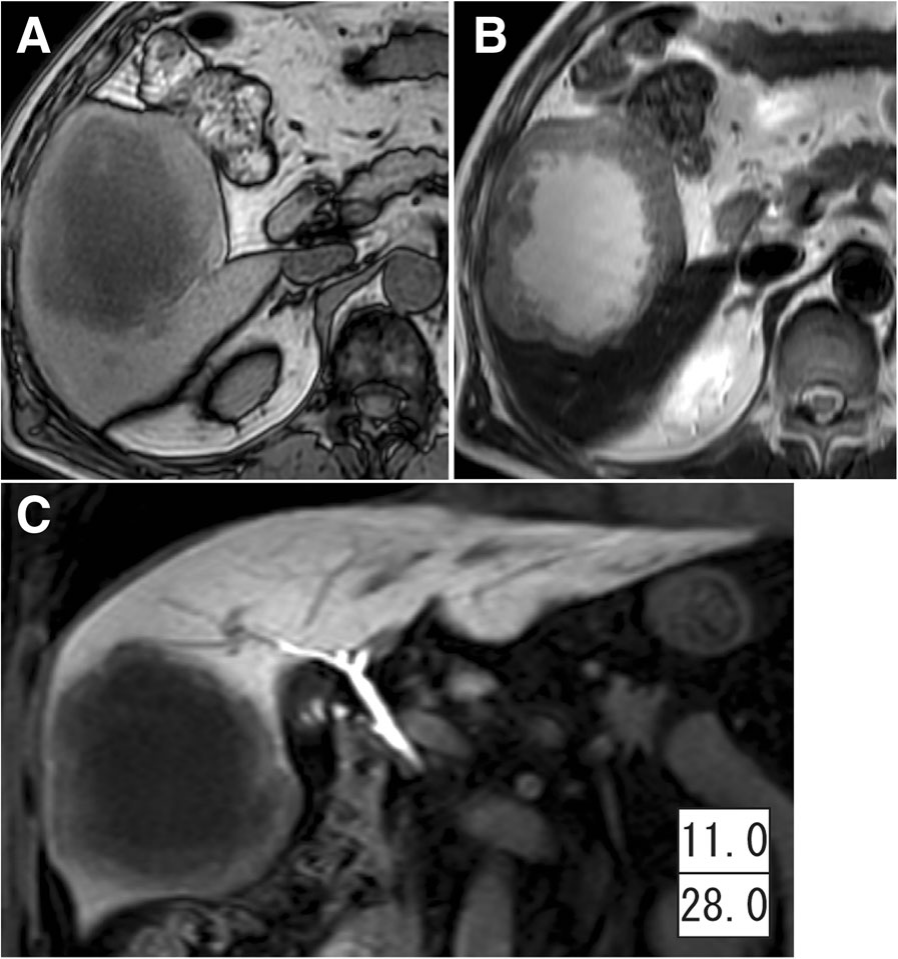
Magnetic resonance imaging demonstrates a large mass with hypointensity on T1-weighted images (a) and hyperintensity on T2-weighted images (b). The tumor exhibits clear hypointensity in the late dynamic and hepatobiliary phases (c). Inset in c shows serum levels of tumor markers at the time (upper: carcinoembryonic antigen [CEA]; lower: cytokeratin 19 fragment [CYFRA])
